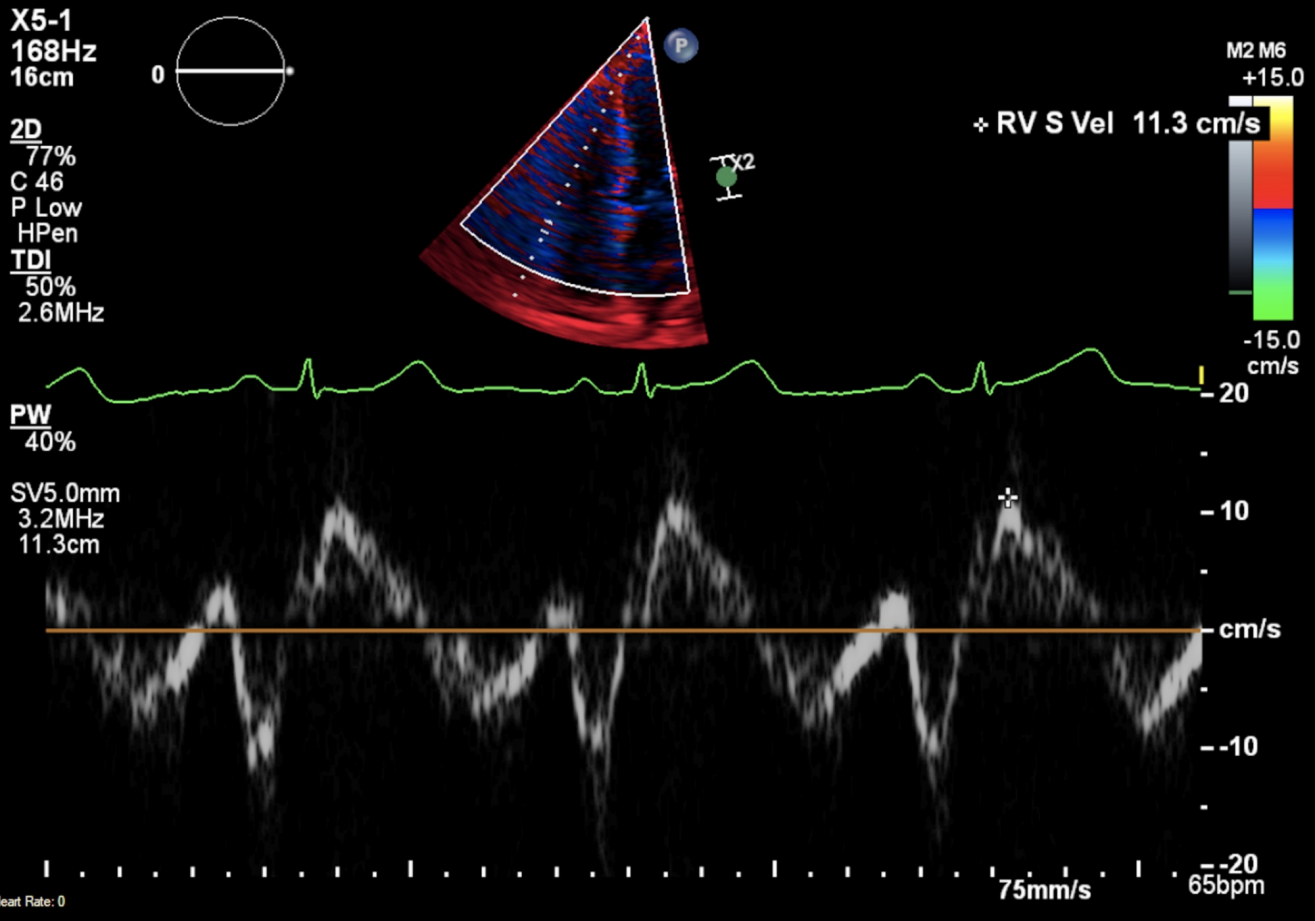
Initial TTE showing RV velocity. TTE: transthoracic echocardiogram; RV: right ventricle
